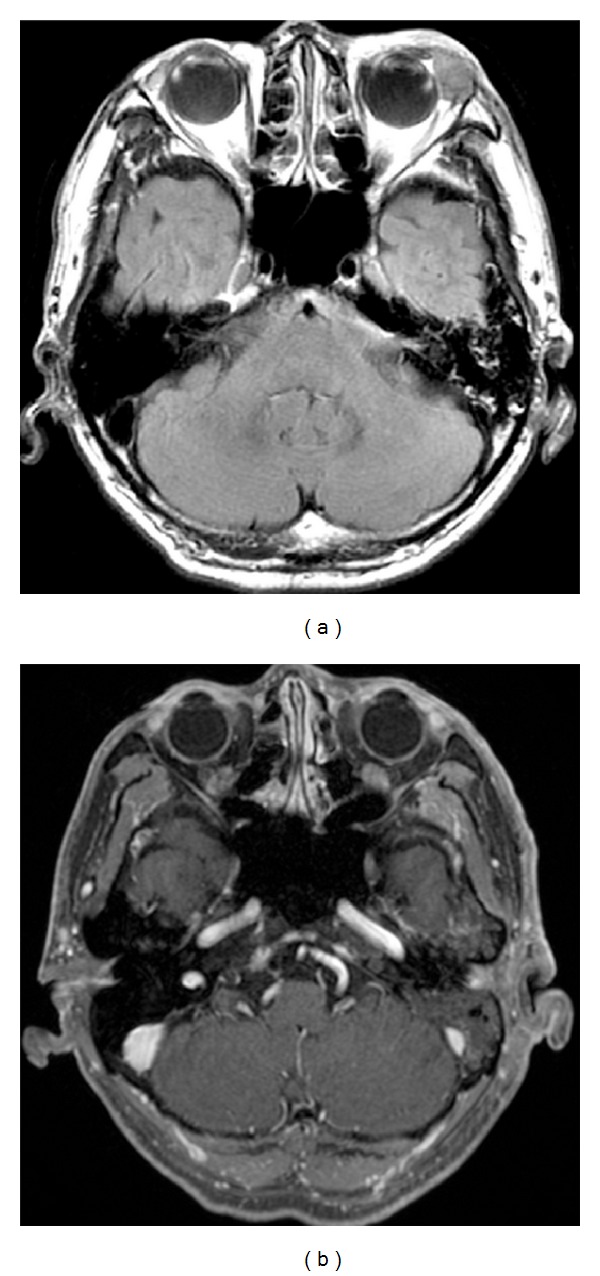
Pretreatment (a) and posttreatment (b) of MRI findings of the left lacrimal gland. After combination therapy, the tumor in the left lacrimal gland was not detectable.
